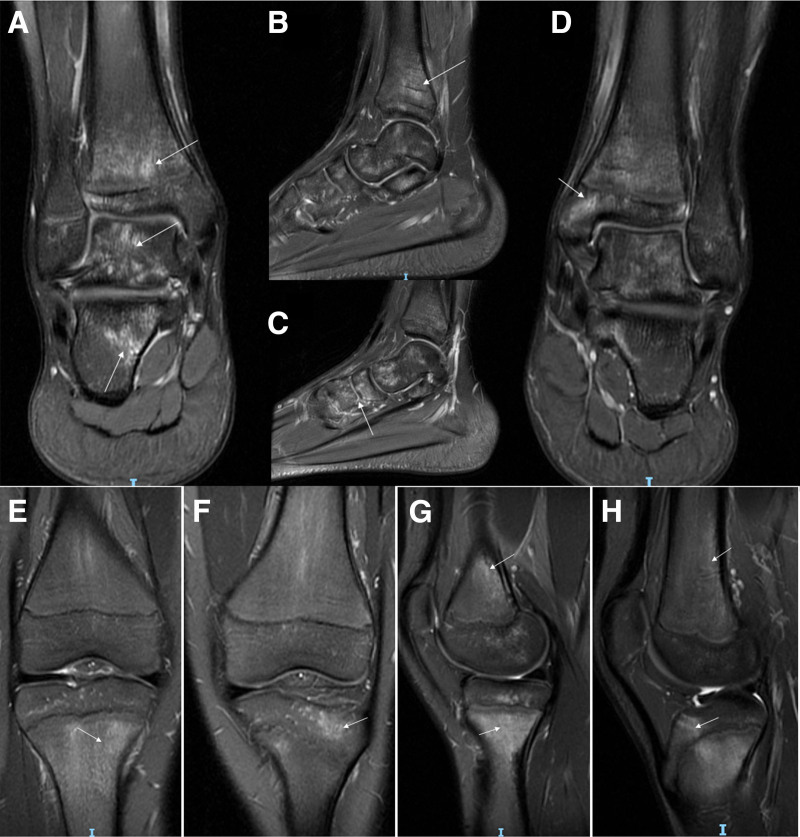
Abnormal signal intensity on MRI of the ankles and knees of a 13-year-old boy. (A and B) The lesions were hyperintense (white arrows) on the coronal and sagittal T2-weighted images of the left ankle; (C and D) the lesions were hypointense on T2-weighted images of the right ankle, involving the tibias, taluses, calcaneus, and dice bones; (E–H) the lesions were hyperintense (white arrows) on the coronal and sagittal T2-weighted images of the right and left knees, involving the femurs and tibias. MRI = magnetic resonance imaging.
